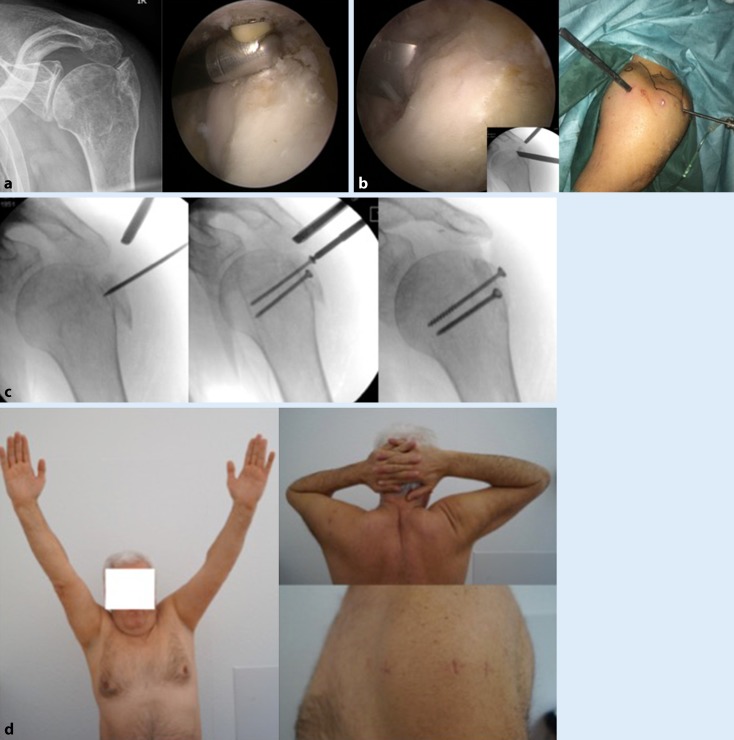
a Posterosuperior malunion of a solid greater tuberosity fracture. Preoperative anteroposterior radiograph (*left*) and intraoperative finding (*right*). b Osteotomy of the fragment (*left*) with corresponding fluoroscopy (*inset*) and external view (*right*). c Under arthroscopic and fluoroscopic guidance, the fragment is reduced and fixed with two 3.5-mm screws. d Functional outcome four months postoperatively
